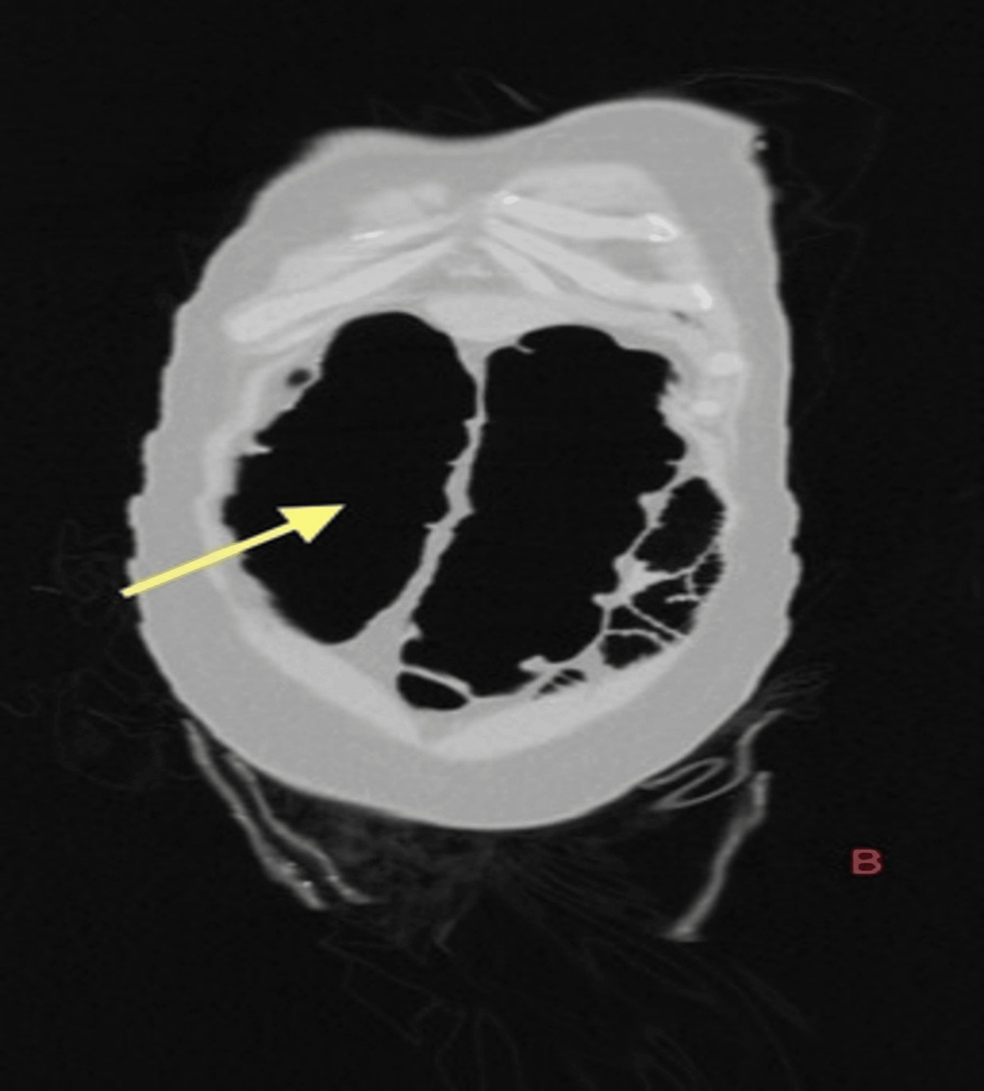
CECT abdomen (coronal plane) showing dilated transverse colon (yellow arrow) of 10.5 cm with normal caliber cecum, ascending colon, and proximal descending colon. The bowel from the distal descending colon appears collapsed CECT: contrast-enhanced computed tomography
